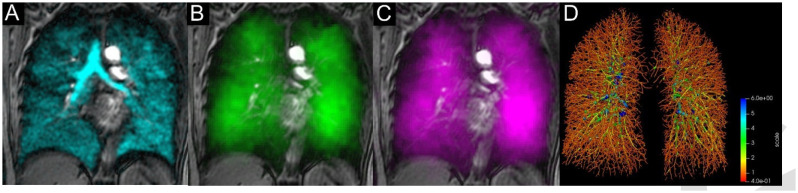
^129^Xe gas-transfer MRI and CT pulmonary vessels in a
